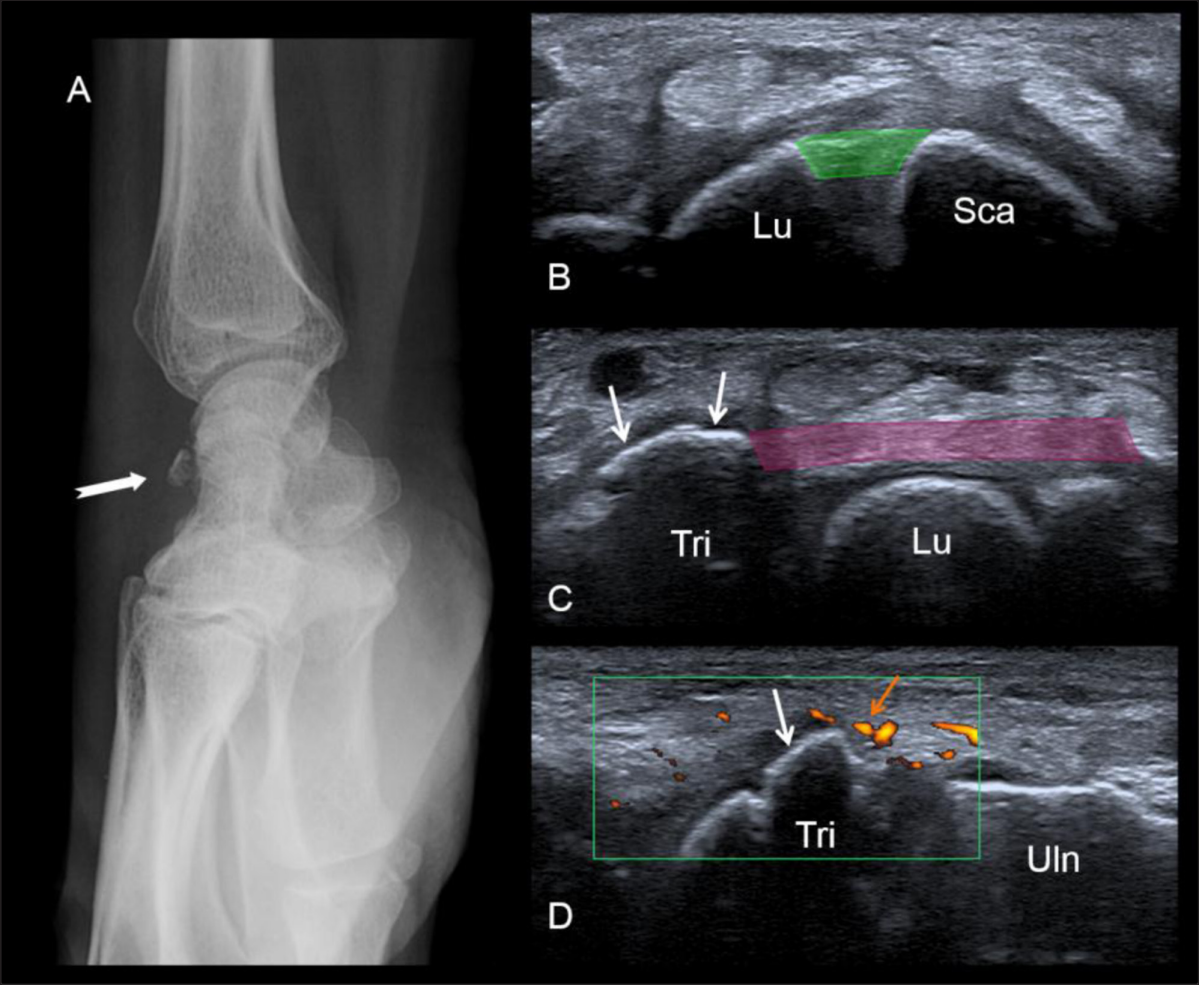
Bony avulsion of the dorsal sling (triquetral insertion). A fracture is seen on the lateral radiograph (A). At the US examination, the dorsal band of the scapholunate ligament (in green) is normal (B). In contrast US shows the bony avulsion of the triquetral insertion of the dorsal radiolunotriquetral ligament (white arrows). See the soft-tissue hyperemia surrounding the avulsion on the long-axis view (orange arrow). Lu = lunate, Sca = scaphoid, Tri = triquetrum, Uln = ulna.
